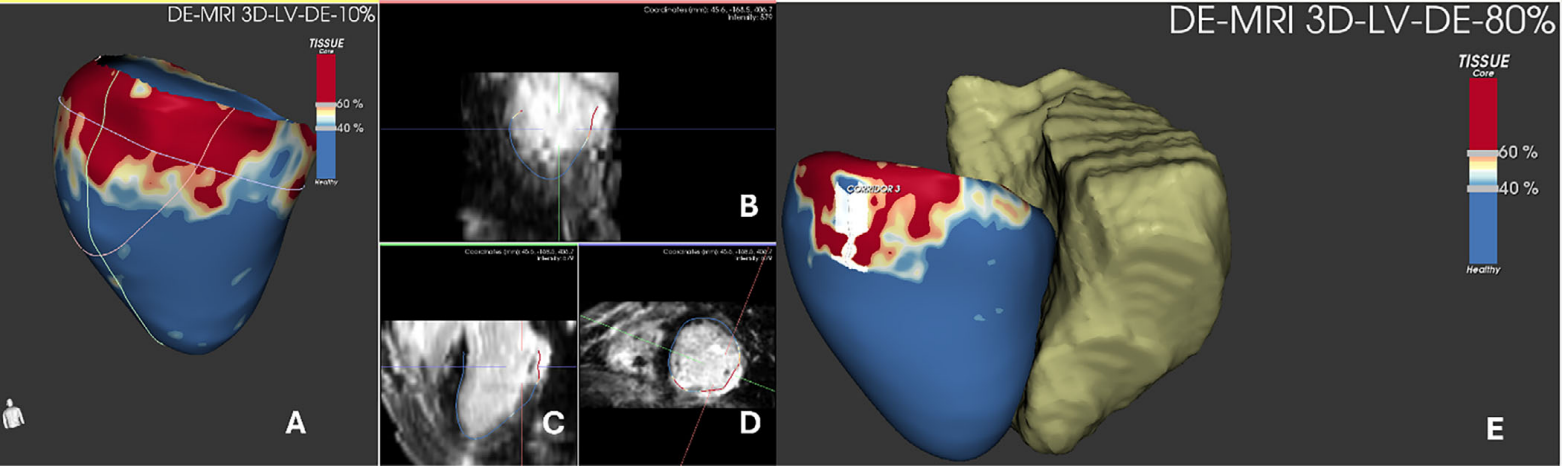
Post‐processing of a LV fibrotic area of the inferolateral wall. (B–D) Multiplanar assessment of LGE via 3D LGE sequence. The post‐processing comprehends a first phase of segmentation followed by a 3D reconstruction based on signal intensity (A). (E) The dedicated software (ADAS 3D, La Galgo Medical, Barcelona, Spain) allows for the integration of another cardiac structure (in this case the right ventricle) from cardiac computed tomography.
